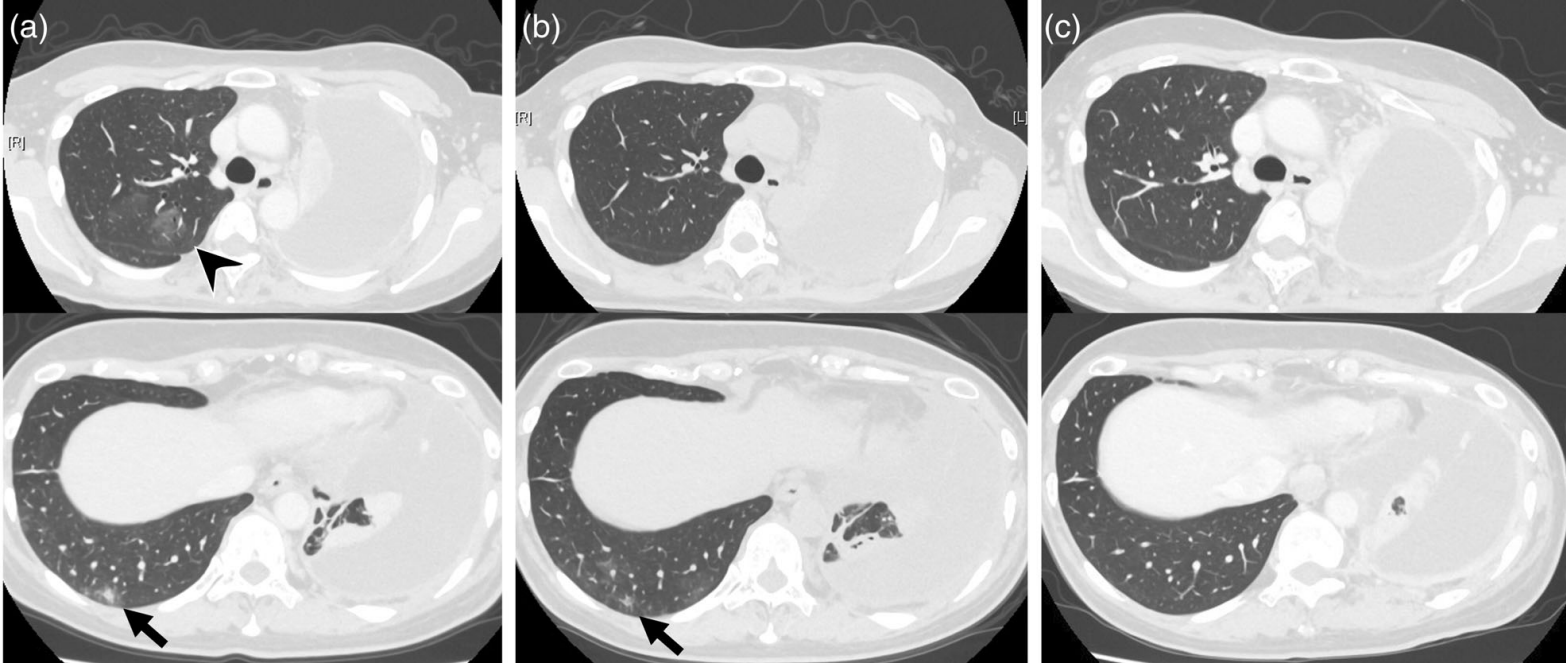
Series of chest computed tomography images. (a) Ground‐glass opacities (GGOs) in the right upper lobe (arrowhead) and a subpleural nodule in the right lower lobe (arrow) after initiation of tepotinib. (b) GGOs disappeared and the subpleural nodule (arrow) appeared to be fading 3 weeks after withdrawal of tepotinib. (c) GGOs and the subpleural nodule disappeared completely 5 months after drug readministration
